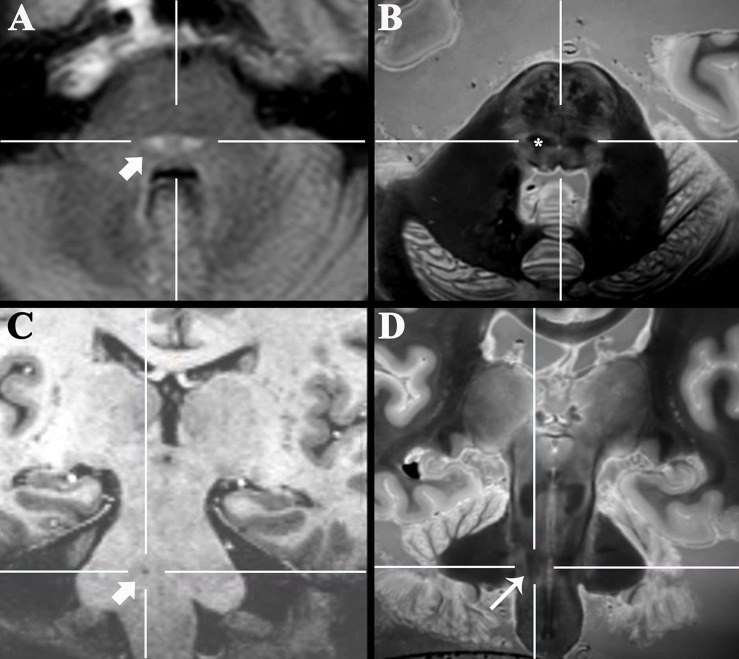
Axial 3-T FLAIR clinical image of a 47-year-old patient with new sensory loss and suspected Multiple Sclerosis (A) demonstrated new bilateral FLAIR-bright demyelinating lesions in the pons (arrow). Coregistered 3D SPACE *ex vivo* image (B) suggested the lesions were in the medial lemniscus (*, labeled unilaterally). Oblique coronal 7-T 3D T1-weighted image (C) of a 77-year-old patient that presented with progressive dysarthria, palatal myoclonus and hypertrophic olivary degeneration (not shown). The precise anatomic location of a tiny T1-dark lacunar infarct in the right pons (arrow) could not be determined even with 7-T MRI. Coregistered 3D SPACE *ex vivo* image at 3-T (D) suggests the lesion is in the central tegmental tract (long arrow).
